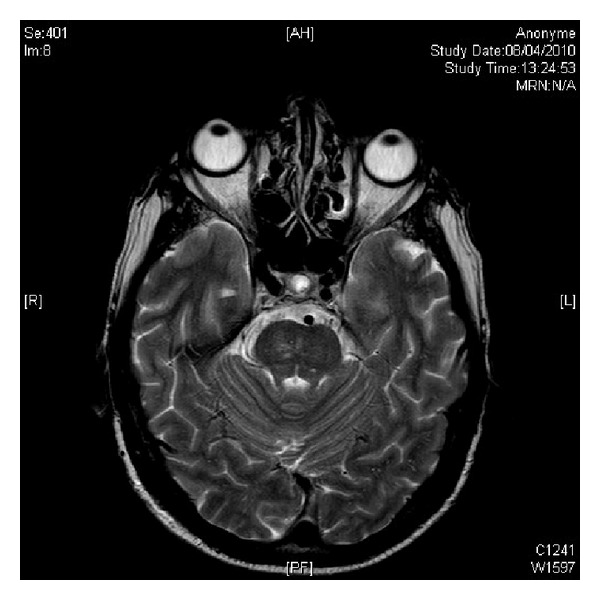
Magnetic resonance imaging (MRI) of the brain without ischemic changes, metastatic lesions, or abnormal enhancement with gadolinium.
